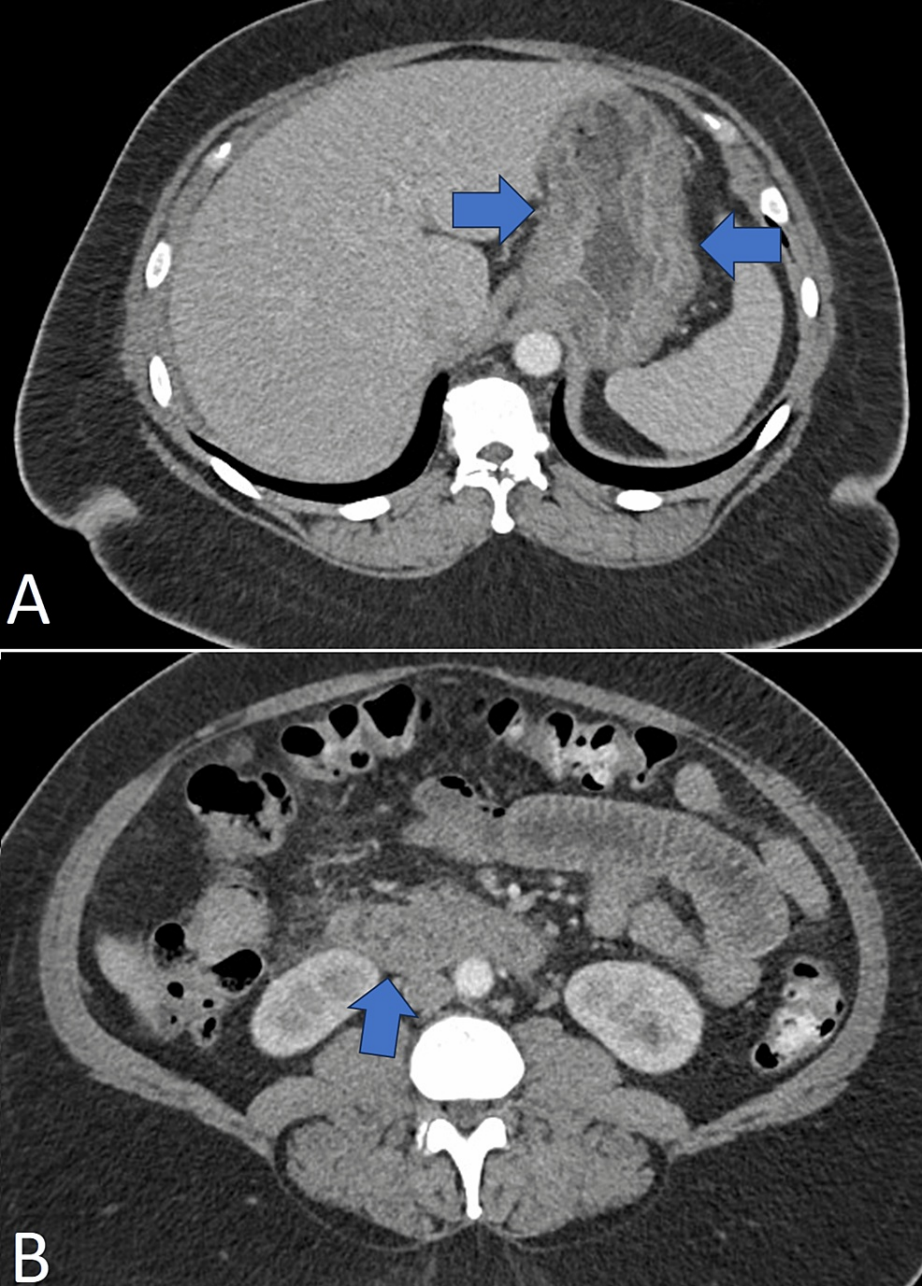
Repeat CT abdomen and pelvis Repeat CT of the abdomen and pelvis on hospital Day 4 showing (A) gastritis (blue arrows) and (B) duodenitis (blue arrow)
